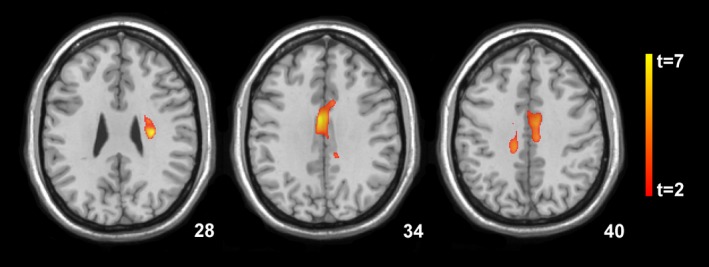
Statistical parametric mapping (*t*) axial maximum intensity projection maps rendered onto a stereotactically normalized MRI scan, showing areas of significant decreases in fractional anisotropy values in a cohort of patients with subarachnoid hemorrhage at 12 months compared to the 3‐week time point (color code, yellow to orange). The number at the bottom right corner of each MRI scan corresponds to the *z* coordinate in Montreal Neurological Institute space.
